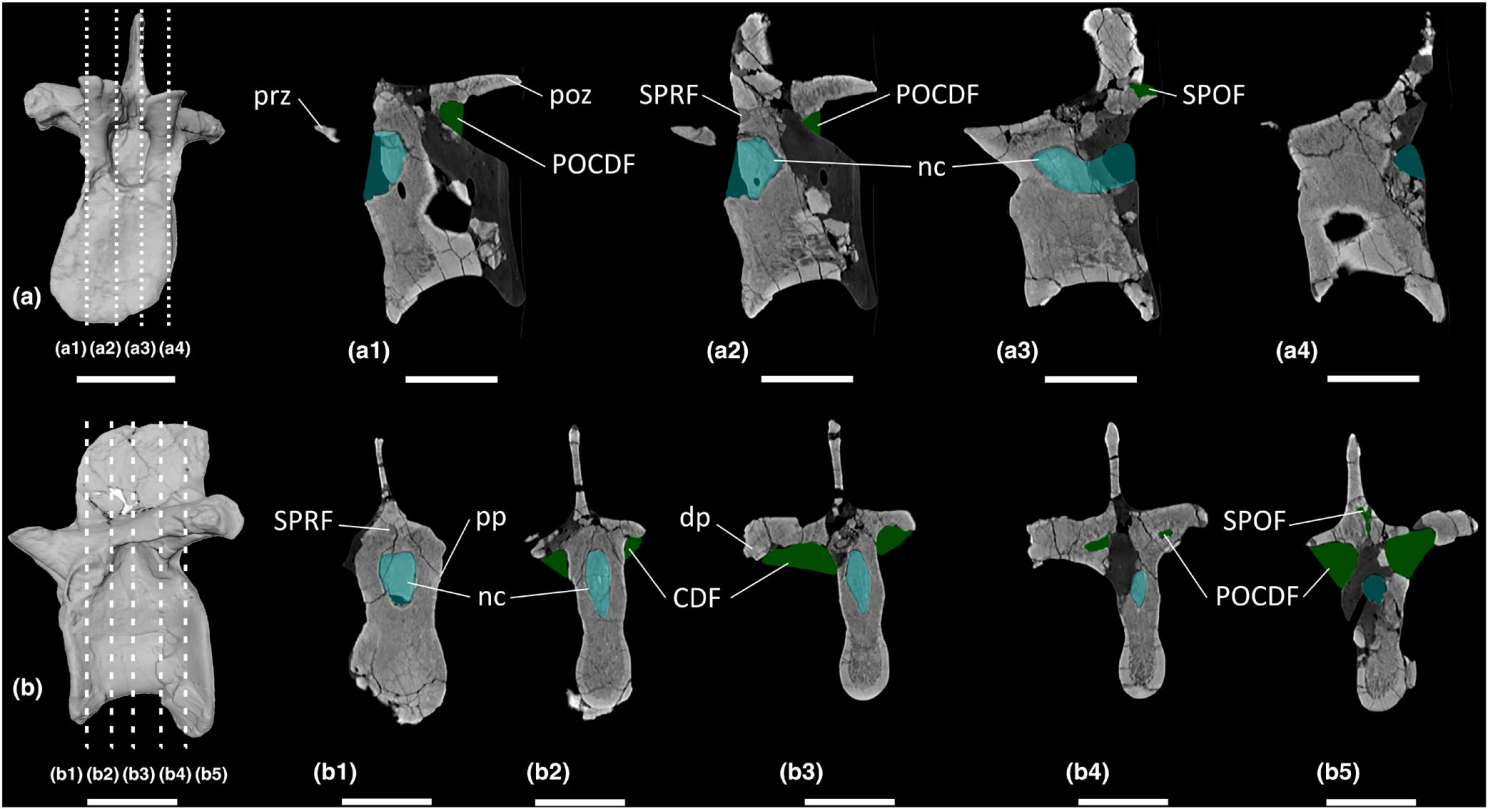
CT scan data of *Plateosaurus longiceps* MB.R.4430 ‘Skeleton C’ middle dorsal vertebra. (a, b) dorsal vertebra 9 MB.R.4430.30; (a) surface scan anterior view, (b) surface scan lateral view; (a1–a4) CT scan slices in parasagittal cross‐section, (b1–b5) CT scan slices in transverse cross‐section. CDF, centrodiapophyseal fossa; dp, diapophysis; nc, neural canal; POCDF, postzygapophyseal centrodiapophyseal fossa; poz, postzygapophysis; pp, parapophysis; prz, prezygapophysis; SPOF, spinopostzygapophyseal fossa; SPRF, spinoprezygapophyseal fossa. Key: Blue highlight, neural canal; green highlight, fossa. Scale bars = 5 cm.
